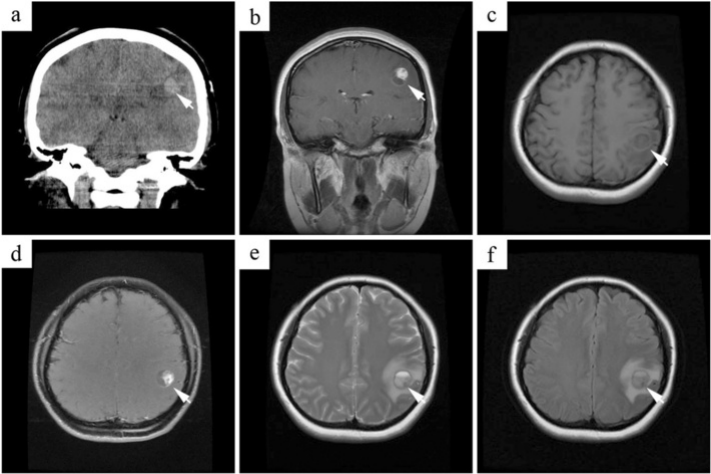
Imaging examination results. a Contrast-enhanced CT reveals a hyperintense oval mass in the left parietal lobe. Post-contrast T1-weighted MRI (b coronal view), T1-weighted MRI (c horizontal view), post-contrast T1-weighted MRI (d horizontal view), T2-weighted MRI (e horizontal view), and FLAIR (f horizontal view) show a well-circumscribed, partially cystic mass with a focally enhancing mural nodule and minimal surrounding edema (both indicated by arrows) as well as a mass effect in the left parietal lobe
